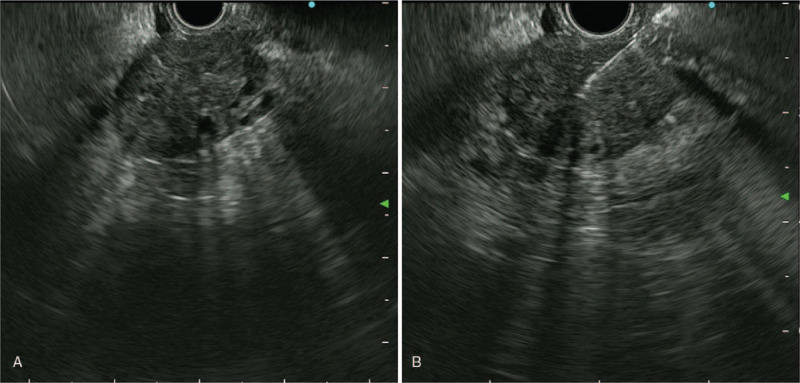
EUS. (A) A 35 mm heterogeneous hypoechoic lesion with anechoic fluid collection in the pancreas tail. (B) Necrotic tissues were aspirated from the pancreatic lesion by EUS-TA. EUS = endoscopic ultrasound, EUS-TA = endoscopic ultrasound-guided tissue acquisition.
